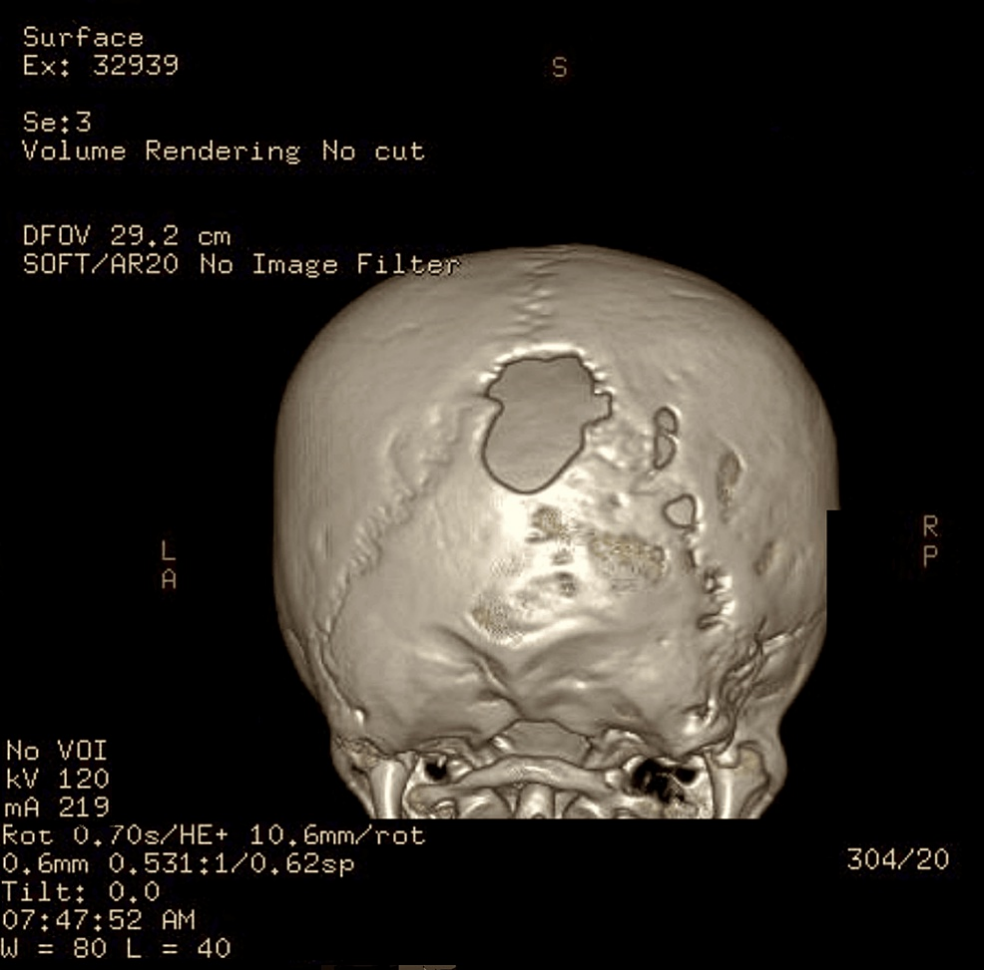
Three-dimensional CT skull showing lambdoid suture bony defect
